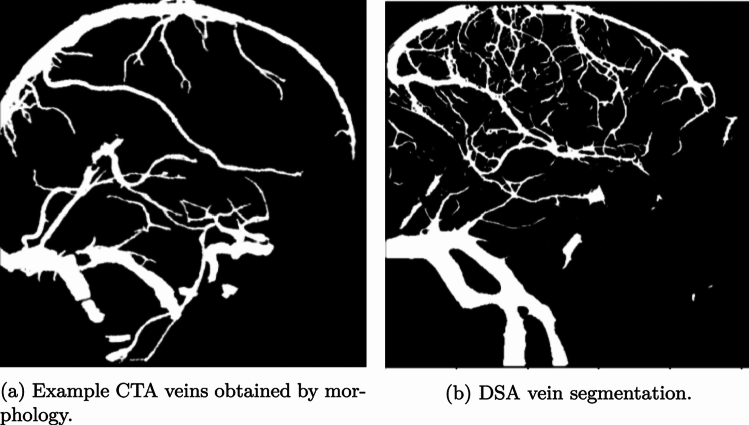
CTA and DSA veins segmentation comparison. The larger vessels typically exhibit less inter-patient variability and are present in both CTA and DSA, thereby making such structures good candidates for an initial learning-based anchoring
